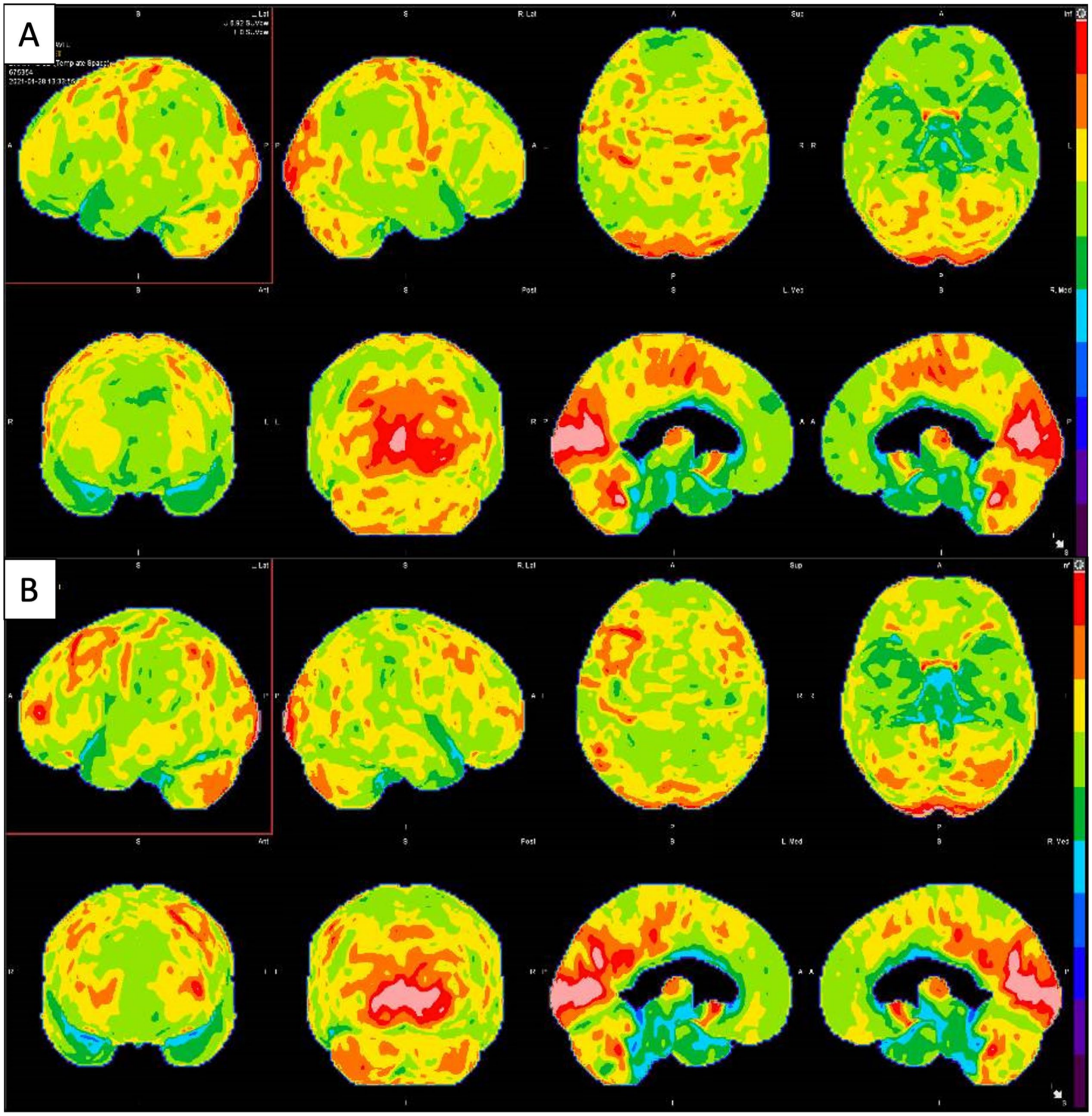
(A) Brain FDG-PET imaging: 3D stereotactic surface projection maps demonstrate mild to moderate hypometabolism in the bilateral parietotemporal regions (slightly more pronounced on the left side), which extends into the frontal lobes. There is accompanying mild hypometabolism in the precuneus and posterior cingulate gyri, regions typically involved early in the setting of Alzheimer’s disease. (B) Second Brain FDG-PET imaging taken 18 months after the first showing improvement in metabolic deficits.
